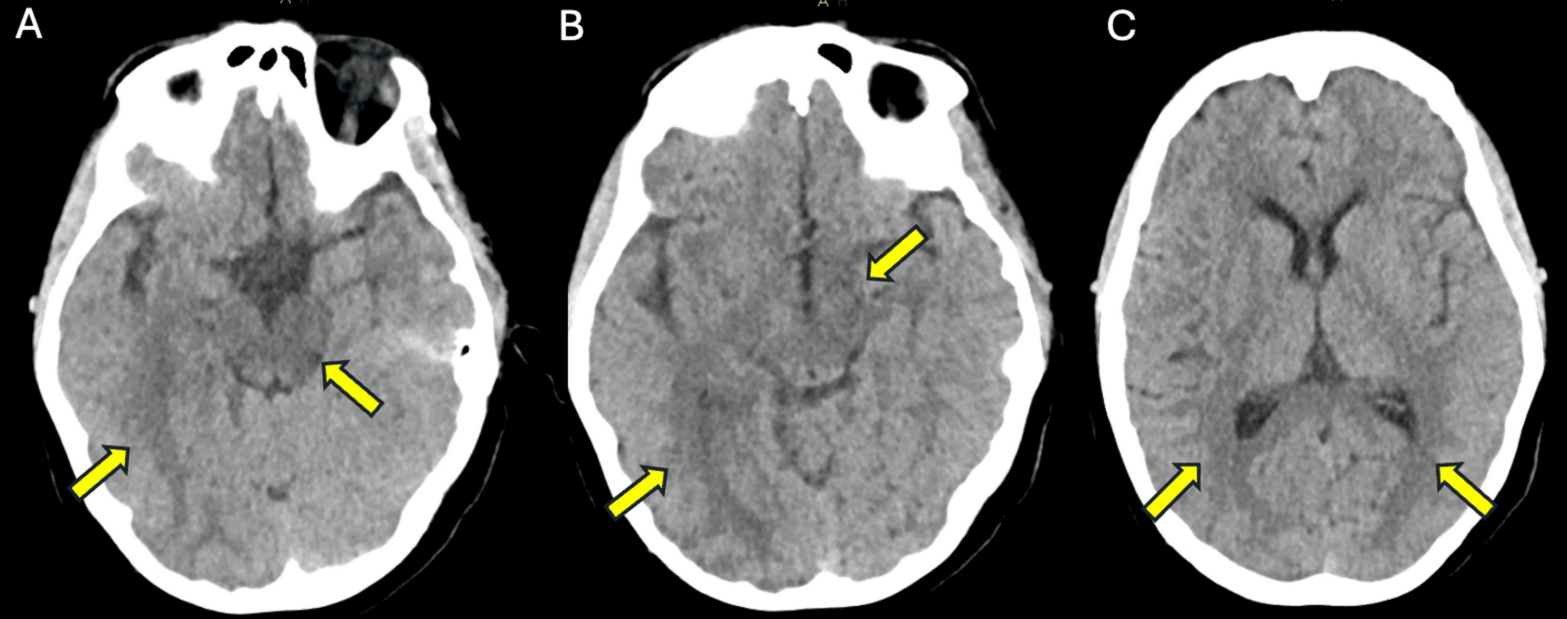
Head CT without contrast showing edema from 6/7 Note: Panels A, B, and C from June 7 progress from caudal to cephalad views, respectively, to show hippocampal and brainstem edema (yellow arrows). No repeat CT scans were performed on June 9.
